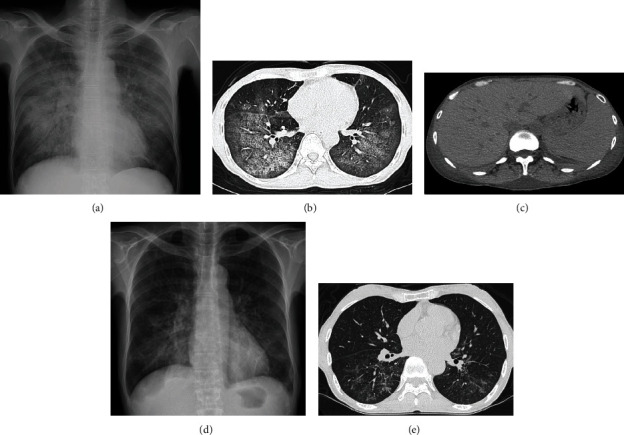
Chest radiography and computed tomography (CT) during hospitalization. (a) Chest radiography on admission showing ground-glass opacity in bilateral lung fields. (b) Chest CT on admission showing bilateral diffuse, hazy ground-glass infiltrates throughout the lungs. (c) Abdominal CT on admission showing mild hepatosplenomegaly. (d) Chest radiography on day 20 showing marked improvement in lung shadowing. (e) Chest CT on day 27 showing marked improvement in lung shadowing.
